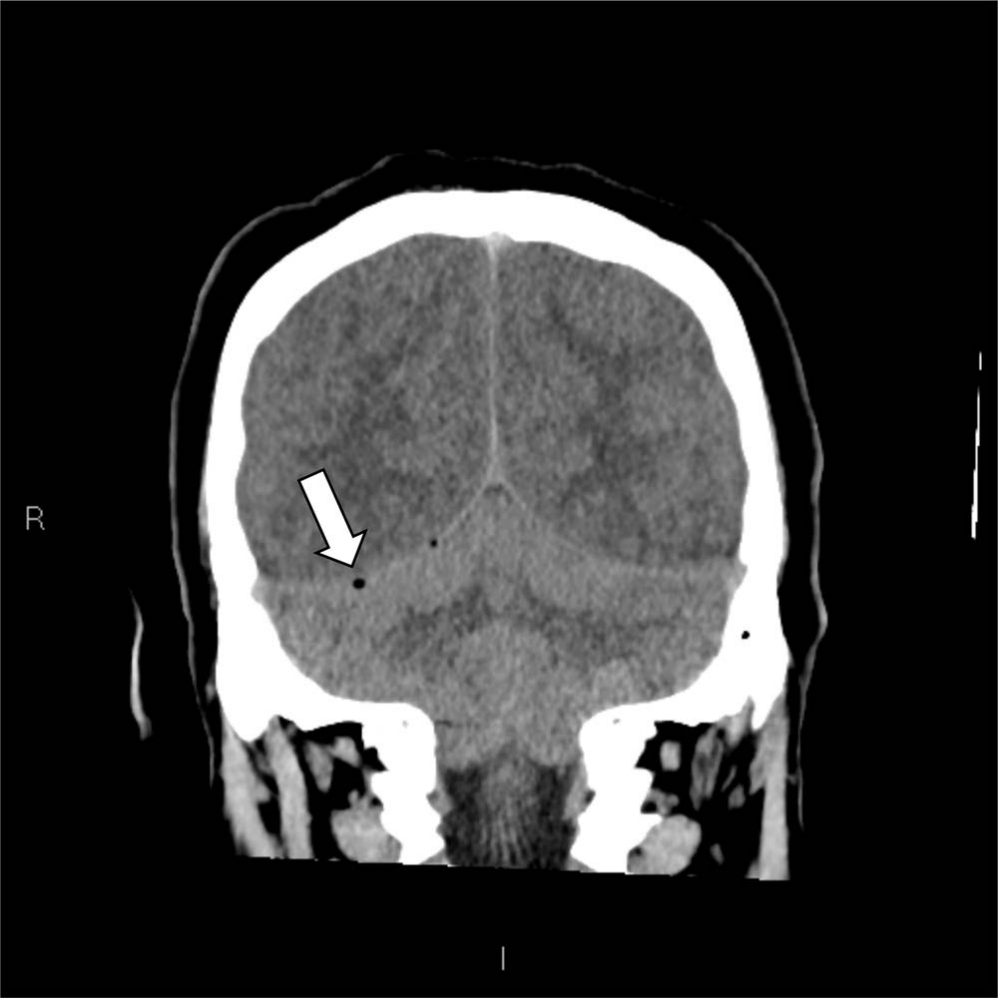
Coronal slice non-contrast CT brain: Cerebellar tonsillar herniation seen along with features of cerebral edema. Note the presence of right-sided pneumocephalus (white arrow) along the tentorium cerebelli.
